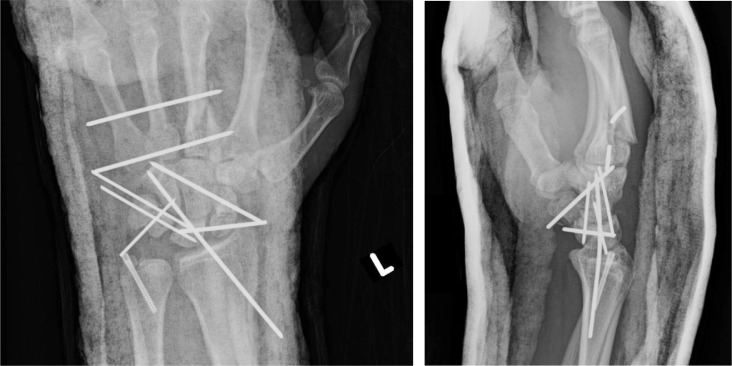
Patient 3: Postoperative radiographs of the left wrist demonstrating pinning across the carpal bones and the third and fourth metacarpal fractures, and screw fixation of the ulnar styloid fracture with improved alignment. Overlying splint material is present.
